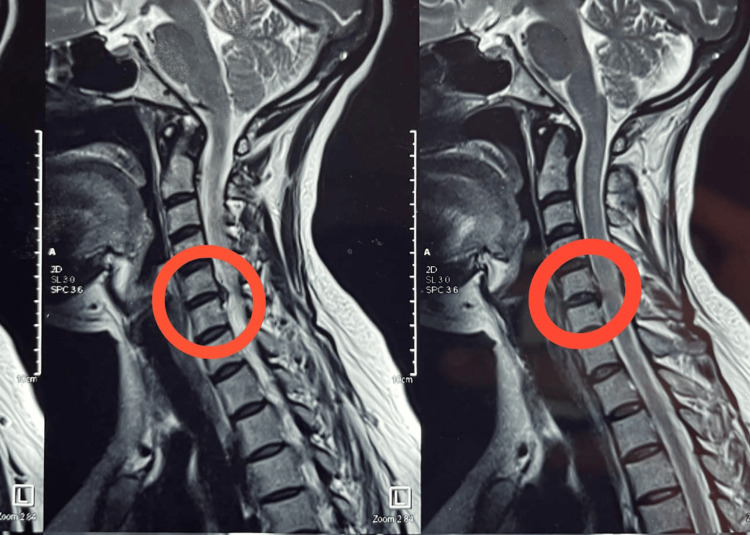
MRI of the cervical spine showing indention to the spinal, which is leading to cervical myelopathy
